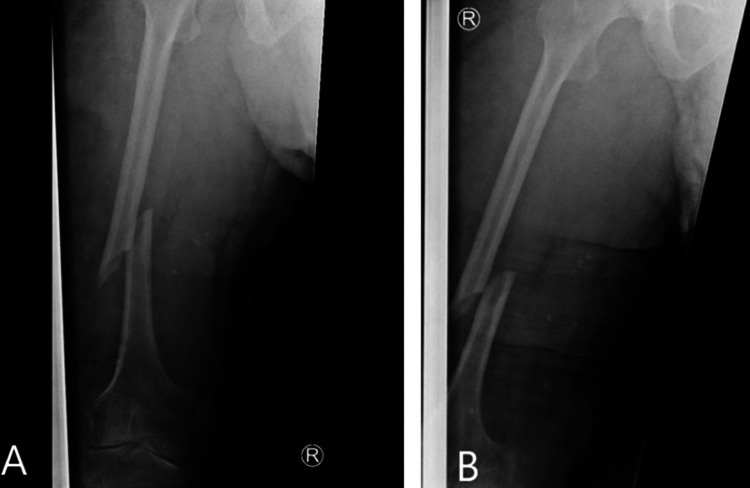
Radiographs showing the initial fracture pattern with anteroposterior (A) and lateral (B) views
